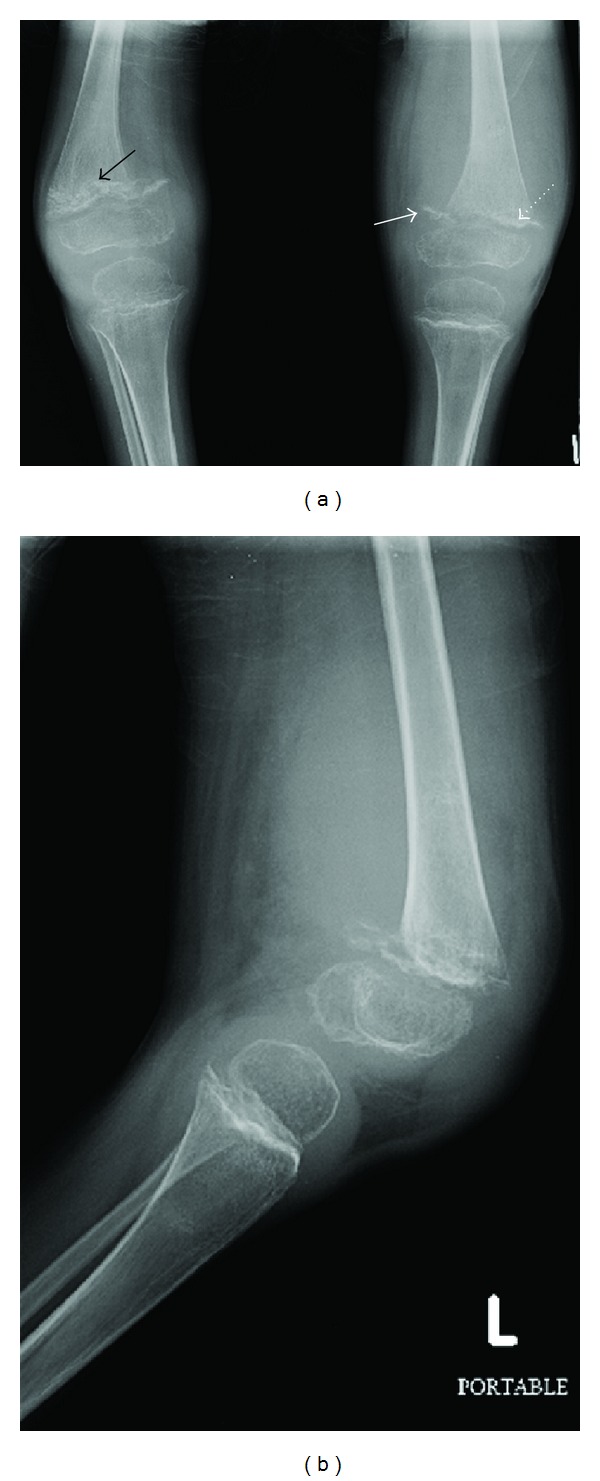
Anteroposterior (a) and lateral (b) X-rays of the knees showing varus deformity. Visible abnormalities include ground glass osteoporosis, thinning of the cortices, lucencies at metaphysis (Trummerfeld zone, black arrow), small corner fractures (Pelkan's spur, solid arrow), and dense zone of provisional calcification in the physis (White line of Frankel, dotted arrow).
